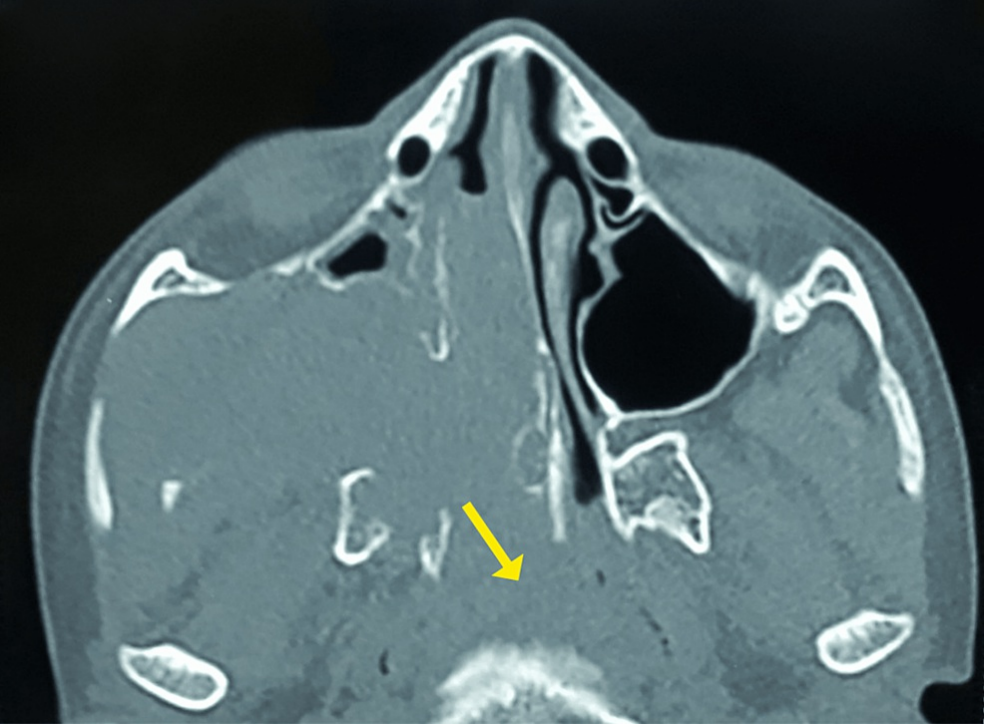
Coronal view showing soft density mass lesion occupying nasopharynx as seen in computed tomography of paranasal sinuses
